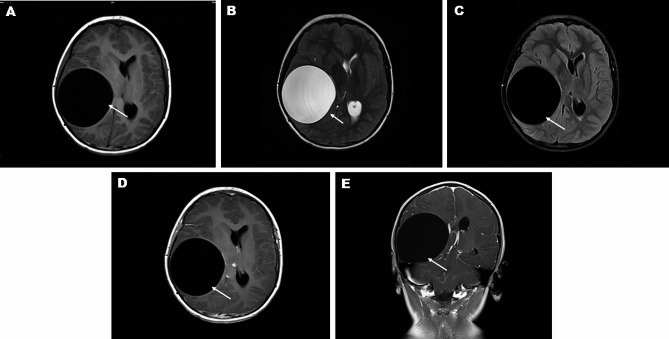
MRI Imaging Features of CCE Lesion (The patient was a 9-year-old boy with a lesion located in the right temporal-parietal lobe, the lesion indicated by white arrows): (A) Hypointense signals in the lesion on cross-sectional T1WI; (B) Hyperintense signals in the lesion on cross-sectional T2WI; (C) Hypointense signals in the lesion on cross-sectional T2-weighted fluid-attenuated inversion recovery (T2W-FLAIR); (D-E) No enhancement observed in the lesion area on contrast-enhanced MRI images
